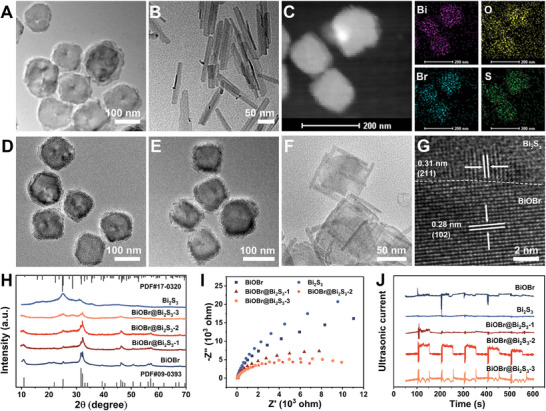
TEM images of A) BiOBr nanosheets and B) Bi_2_S_3_ nanorods. C) HAADF image of S‐scheme BiOBr@Bi_2_S_3_ nanoheterojunctions and the corresponding elemental mapping images. D–F) TEM images of BiOBr@Bi_2_S_3_‐1 (D), BiOBr@Bi_2_S_3_‐2 (E), and BiOBr@Bi_2_S_3_‐3 (F). G) HRTEM image of the BiOBr@Bi_2_S_3_‐2. H–J) XRD analysis (H), EIS spectra (I), and transient ultrasonic current spectra (J) of BiOBr, Bi_2_S_3_, BiOBr@Bi_2_S_3_‐1, BiOBr@Bi_2_S_3_‐2, and BiOBr@Bi_2_S_3_‐3.
